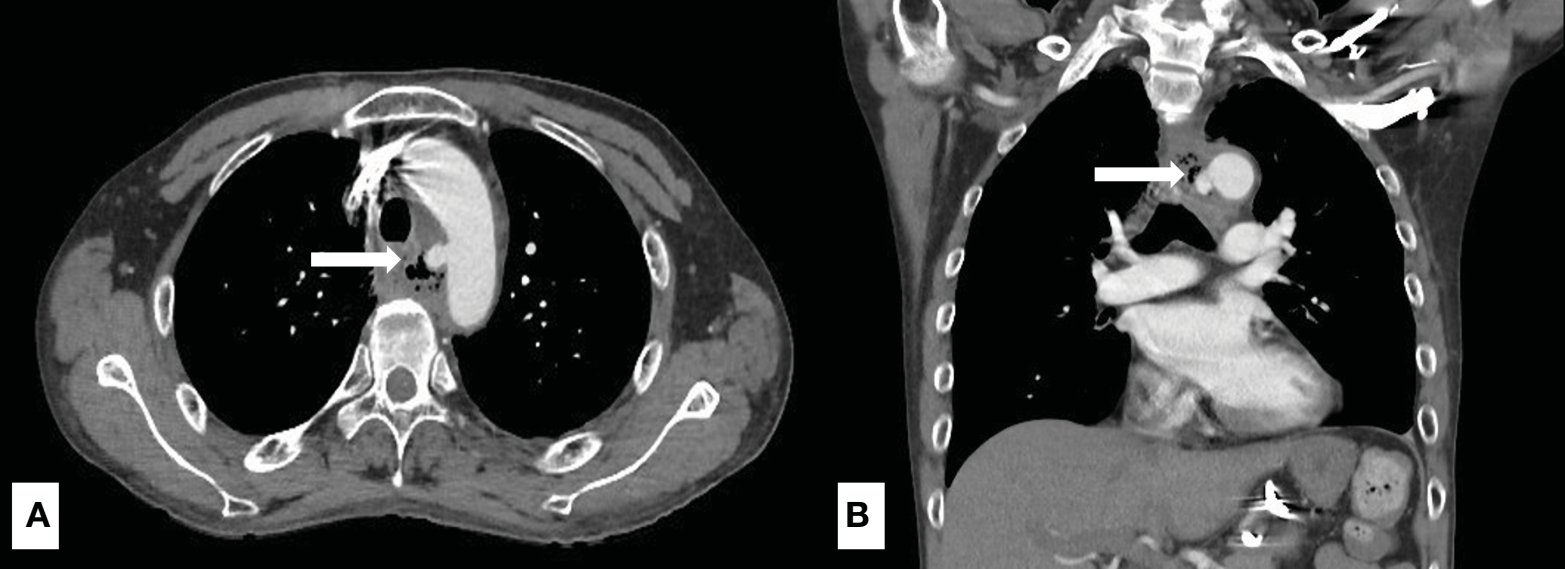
A 45-year-old male diagnosed with esophageal cancer post concurrent chemoradiotherapy. The computed tomography angiography of aorta revealed extravasation from the aortic arch abutting to esophagus (white arrow) with high suspicion of aorto-esophageal fistula in the axial view (A) and the coronal view (B).
